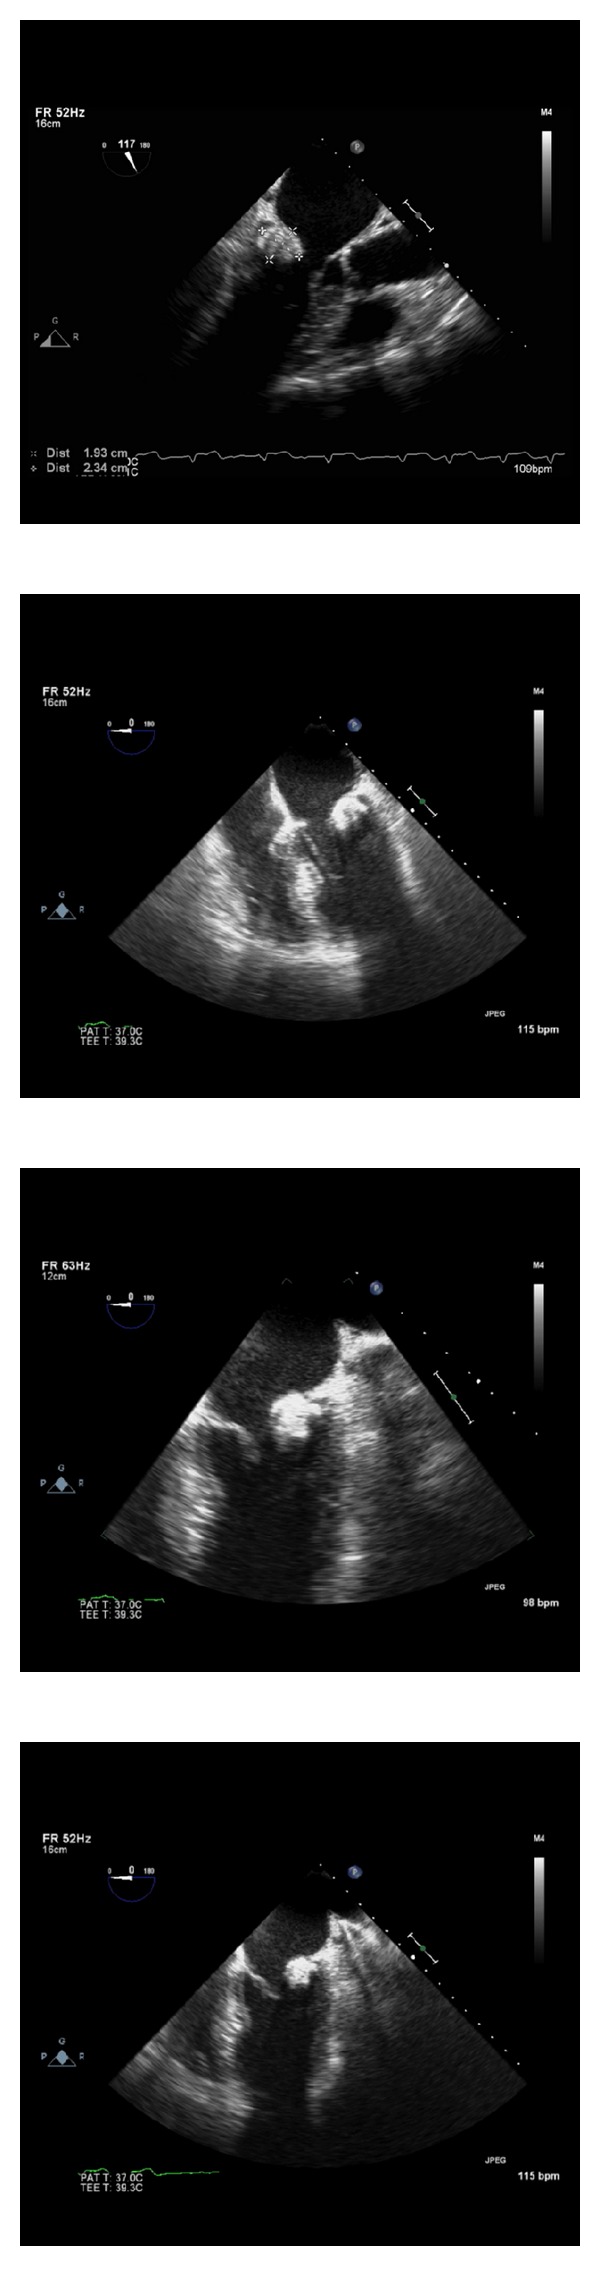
Transesophageal echocardiogram views. Four standard views that show a well-circumscribed echogenic mass that measures 19 × 23 mm and appears to be attached to mitral annulus and extending into the posterior mitral valve leaflet.
